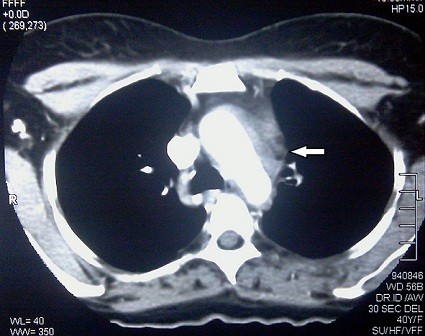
Contrast CT thorax demonstrating the thickening of aortic wall. (White arrow).
